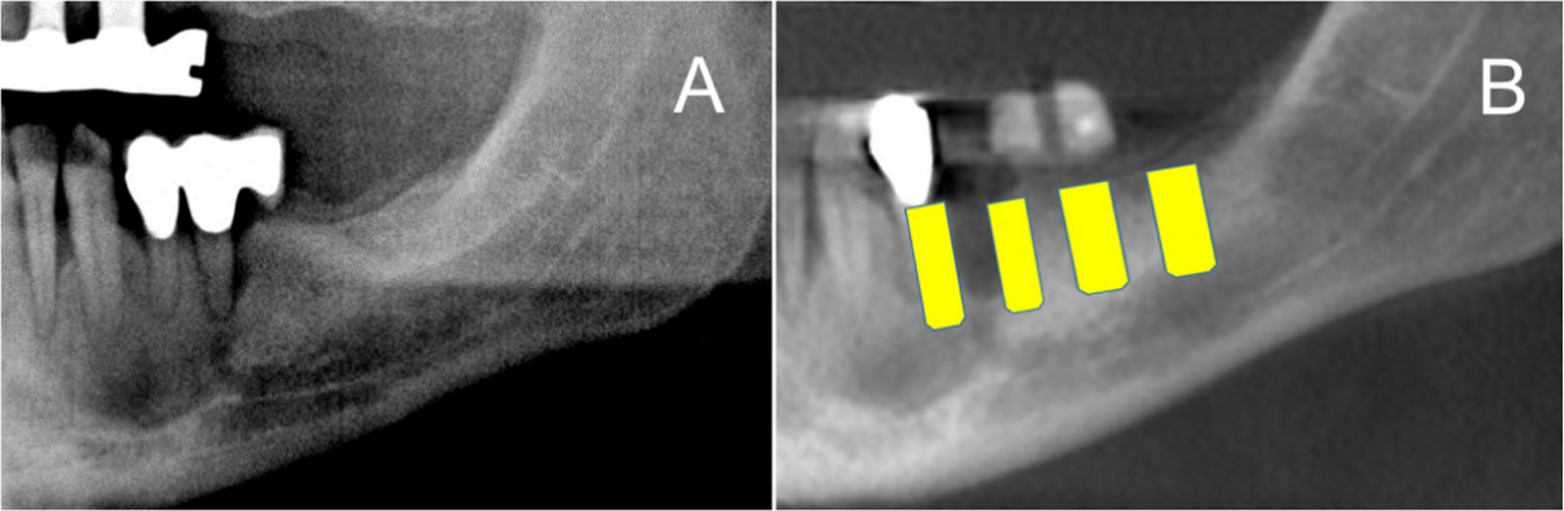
A Initial panoramic radiography of the left mandible; B the determination of the planned implants in regions 34–37 using an orientation template
